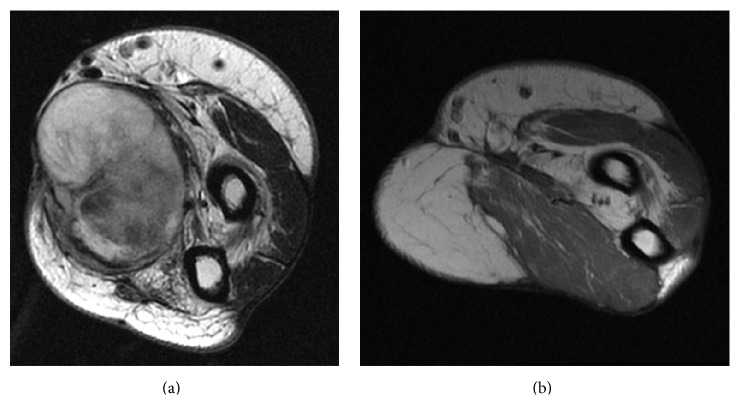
Preoperative T2 axial MRI image showing a heterogenous, subfascial mass (a) compared with postoperative T1 axial MRI image showing no evidence of residual mass (b).
